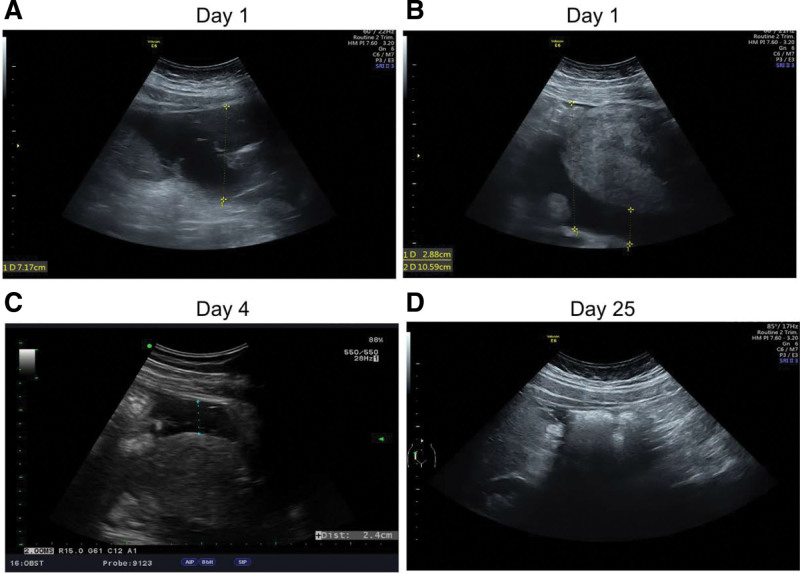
Ultrasound examination of bacterascites. (A) Ascites at the right upper quadrant of the abdomen on d 1. The largest pocket was 7.1 cm. (B) Ascites at the cul-de-sac on day 1. (C) Post-admission d 4. Ascites decreased a lot. (D) Post-admission d 25 (8 d after discharge). No ascites were noted.
